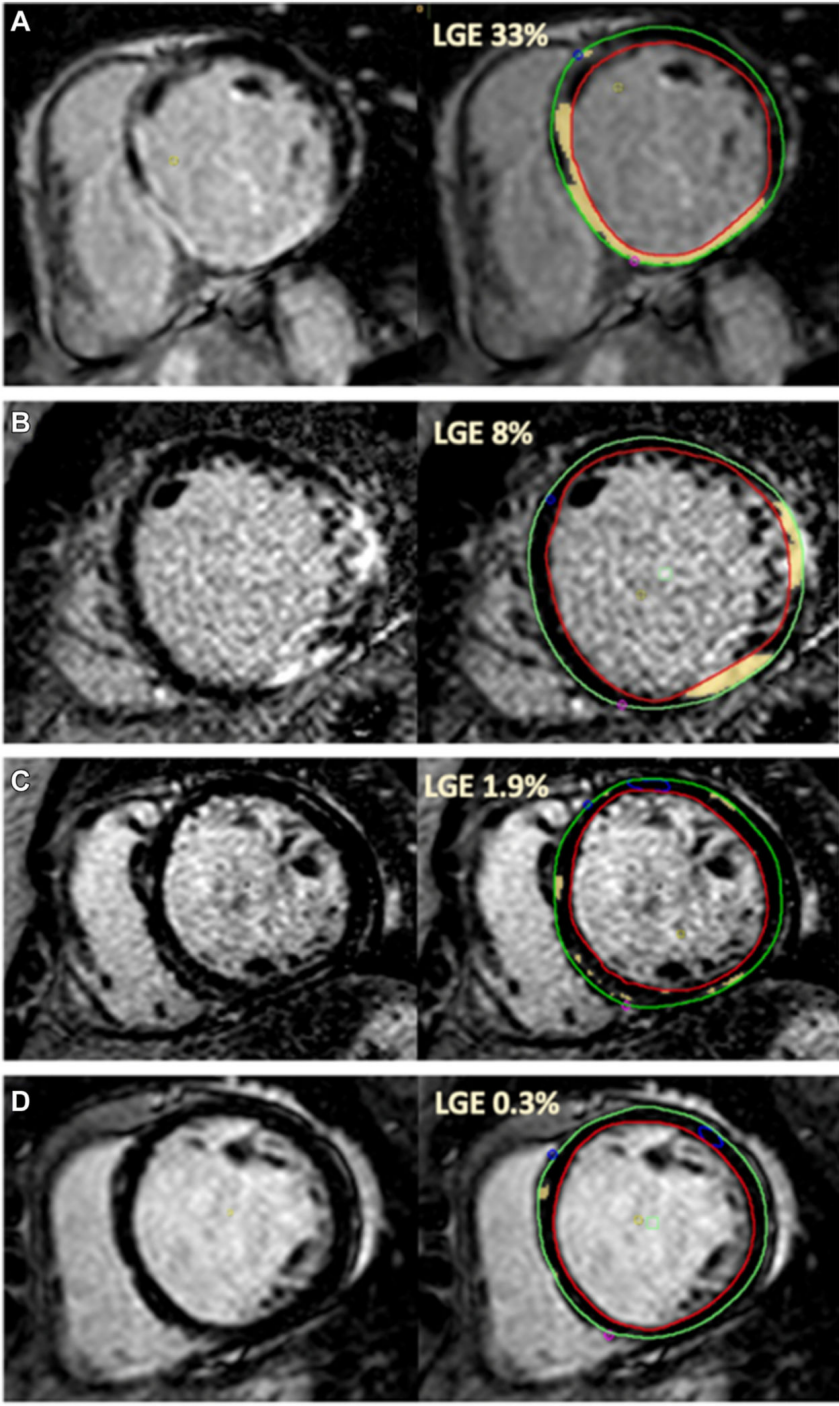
Representative MRI Images. Shown are representative MRI images of patients in this study. A and B: Patients with significant LGE burden who received ICD therapies. C and D: Patients with minimal LGE who received no ICD therapies. LGE % represents LGE burden for the entire left ventricle. Left ventricular walls are outlined with *green* and *red* contours; LGE distribution is highlighted in *yellow*. ICD = implantable cardioverter-defibrillator; LGE = late gadolinium enhancement; MRI = magnetic resonance imaging.
